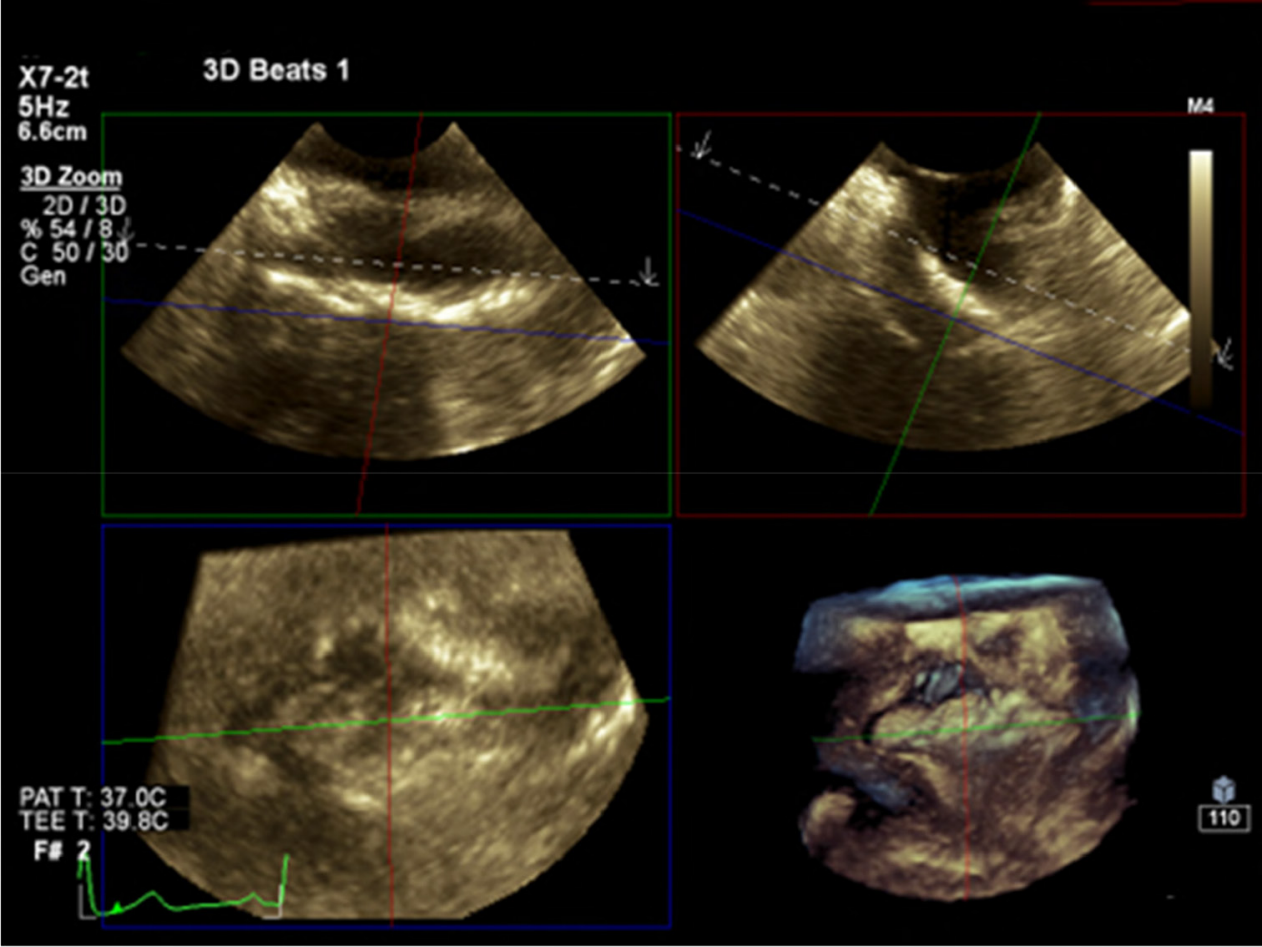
The 3D transesophageal echocardiography shows a multiplanar reconstruction of the elongated irregular hypermobile echodense mass in the right atrium.
